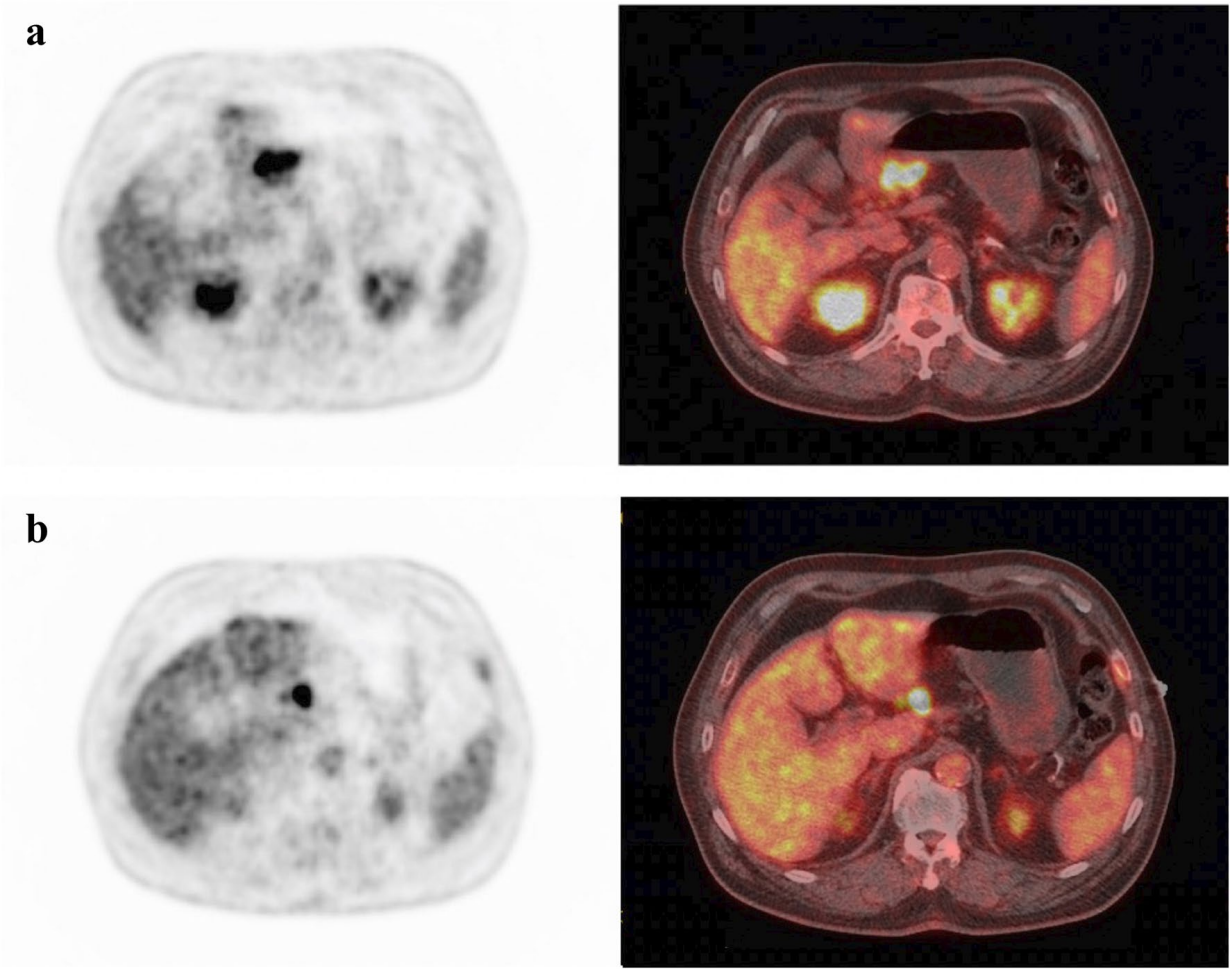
Preoperative FDG-PET/CT of a 72-year-old man showing FDG uptake (a T-SUVmax, 11.53; b N-SUVmax, 12.29). This patient underwent subtotal gastrectomy and stage III gastric cancer was confirmed (pT3N2M0 pStageIIIA, well-differentiated tubular adenocarcinoma).
